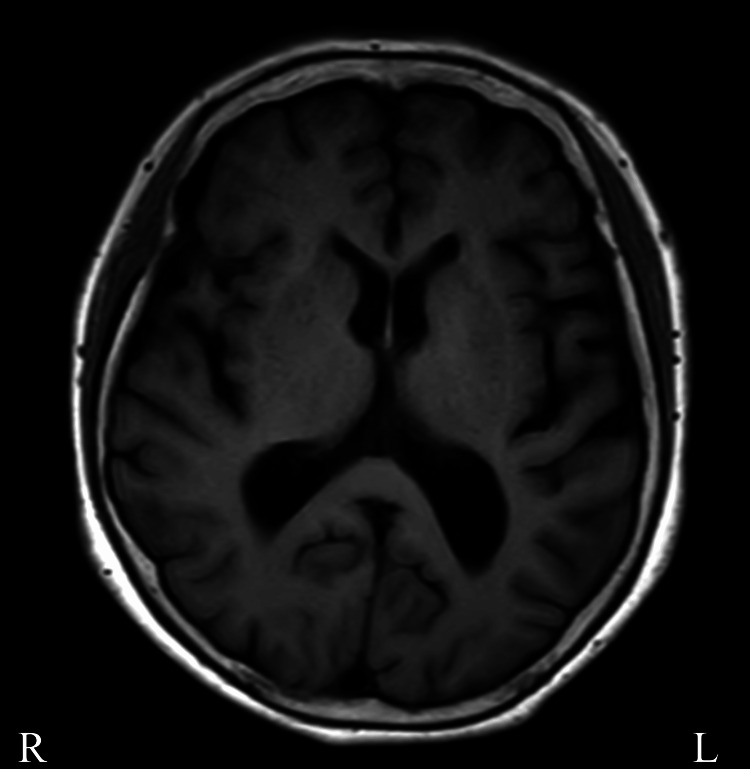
MRI findings Fluid attenuated inversion recovery (FLAIR) image demonstrating prominent atrophy in the left frontal and parietal lobes, with widening of the sulci and ventricular enlargement.
